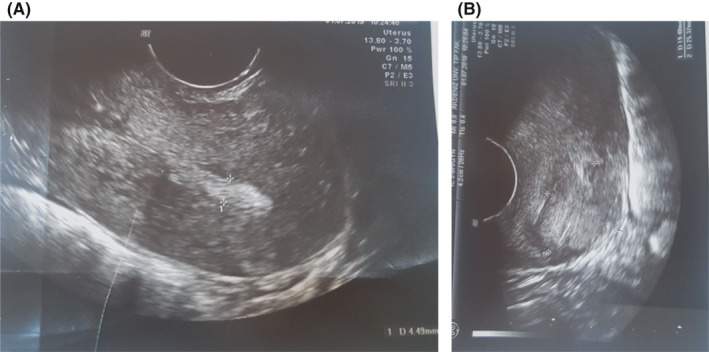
A, Normal endometrial cavity was seen in transvaginal ultrasonographic examination. B, On transvaginal ultrasonographic examination, 26 × 25 mm mass lesion was diagnosed in the old cesarean scar line of the retroverted uterus
